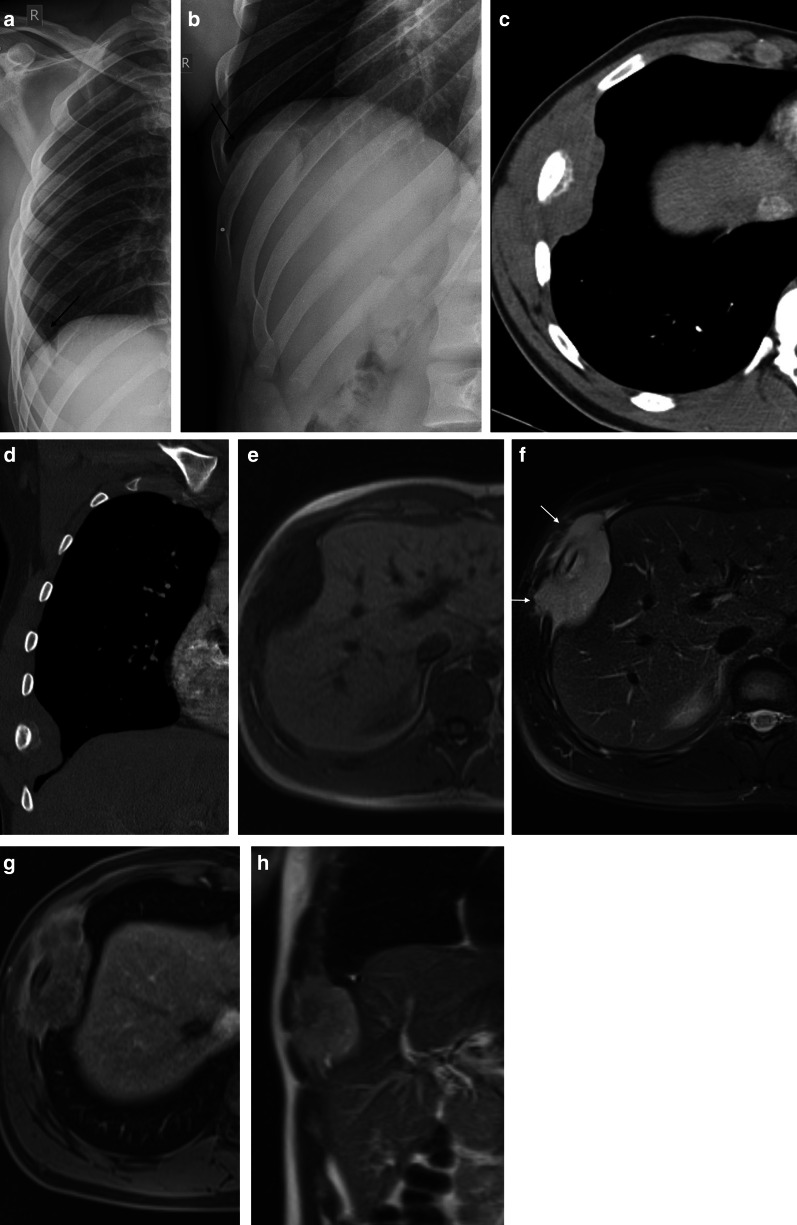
Diagnosis: Ewing sarcoma. Technique: standard chest radiography and contrast-enhanced chest CT. Description: A 23-year-old man was referred for a chest radiograph due to pain at the right hemithorax. The postero-anterior chest radiographs (a, b) show an opacity (arrow) projecting at the level of the 7th right rib. An external radio-opaque marker was placed on the painful area. The additional contrast-enhanced CT further characterizes the mass encasing the 7th right rib with a locoregional aggressive periosteal sunburst type reaction and a surrounding soft tissue component (c, d). On the axial T1-weighted MR image (e) the lesion has an isointense signal to muscle and a high signal intensity on the axial FS T2-WI (f) with invasion of the muscles of the chest wall (arrows). After intravenous contrast administration, the lesions show a heterogeneous enhancement (g), predominantly at the periphery of the lesion. The coronal T2-weighted MR image (h) shows the extrinsic impression of the lesion on the liver
